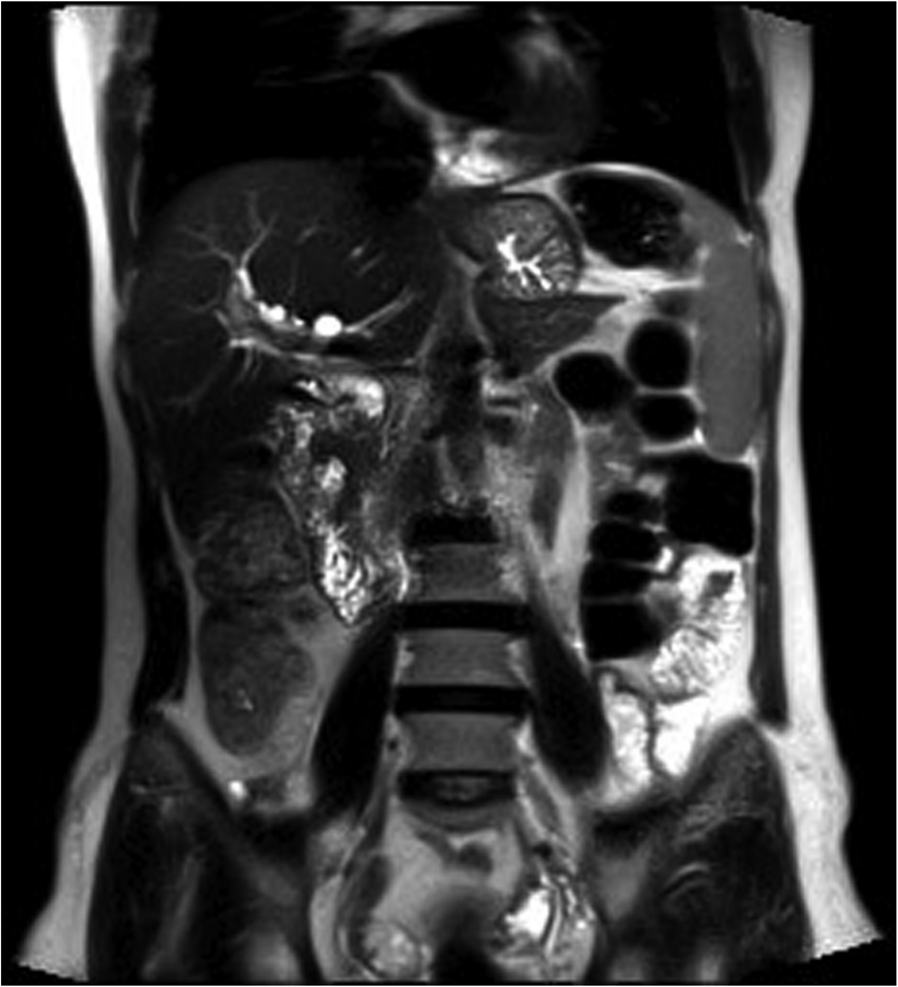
T2-weighted MRI shows the hypointense tumoral lesion in the periampullary region which has a crescent-like shape
